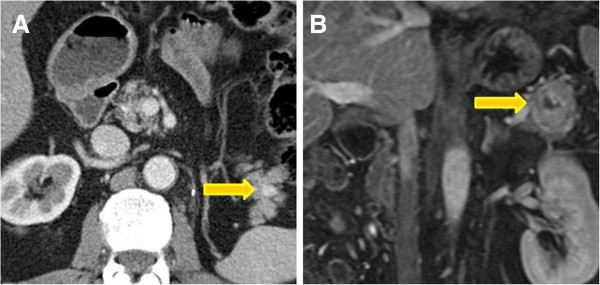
Solitary lesions. Two examples of solitary spherical tumors of the pancreatic tail, respectively on contrast-enhanced CT (A) and MRI (B) images, with the typical appearance of a predominantly solid, well-defined mass with smooth borders.
